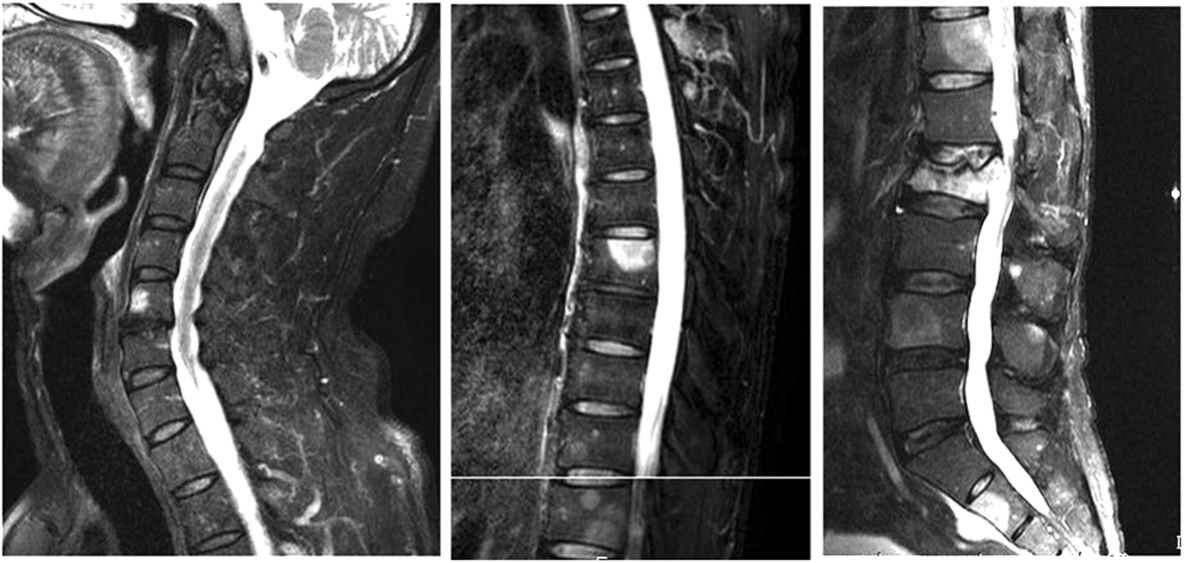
Magnetic resonance imaging shows high signal intensity on a T2-image with fat suppression in multiple vertebrae. Pathological fracture is evident in the second vertebra. Extraskeletal extension is visible in the eleventh thoracic vertebra, second lumbar vertebra and second sacral vertebra.
